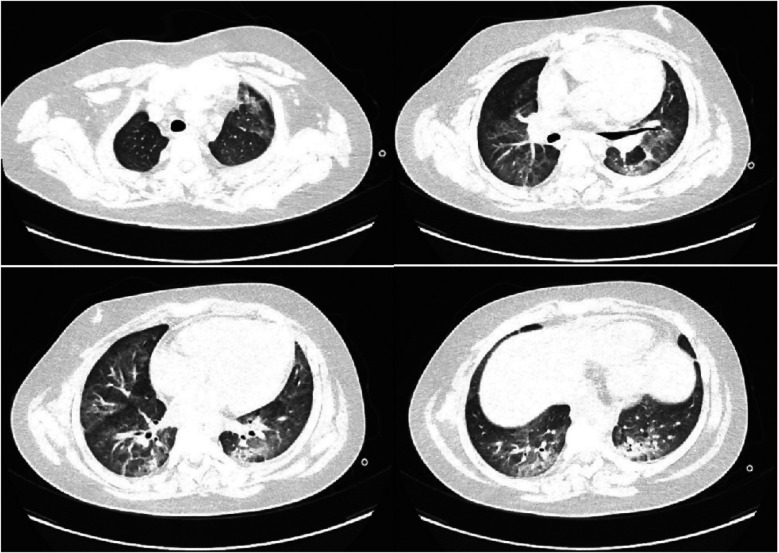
Chest CT findings of the child on day 28 of hospitalization.
